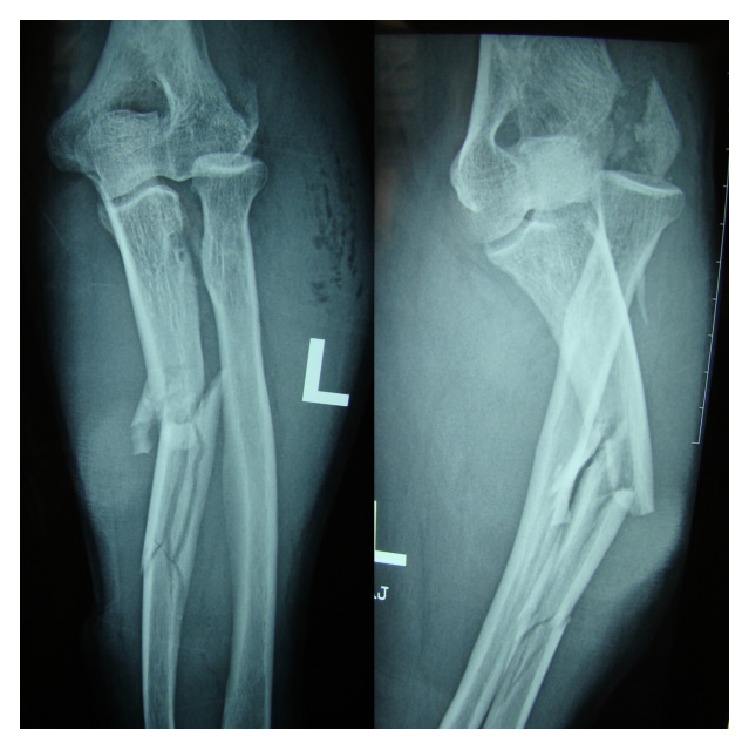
AP and lateral radiographs of the left forearm showing the Monteggia type 2 variant with fractured lateral humeral epicondyle.
